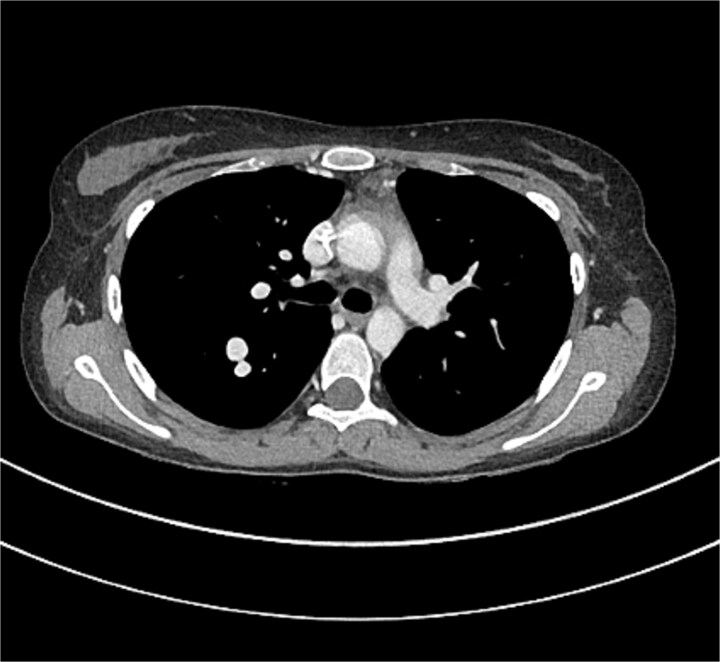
CT of the chest post-receiving chemotherapy by three months.
